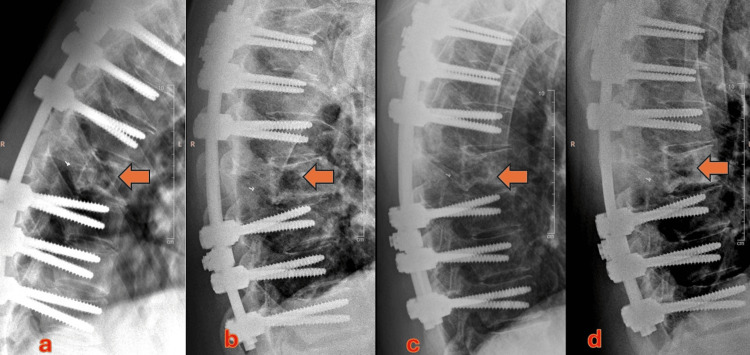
a) Before starting sclerotherapy; the arrow depicts the vertebra with lytic changes. b) Radiograph after two sessions of sclerotherapy with sodium tetradecyl sulfate (STS) foam showing not obvious improvement; the arrow depicts the area of lysis. c) Obtained one month after the first injection of STS + gelatin sponge slurry; shows early sclerotic changes within the previously seen lytic area, marked by an arrow. d) Obtained one month after three sessions of sclerotherapy with STS+ gelatin sponge slurry. A marked increase in the bony sclerosis within the lytic lesion is shown by the arrow.
